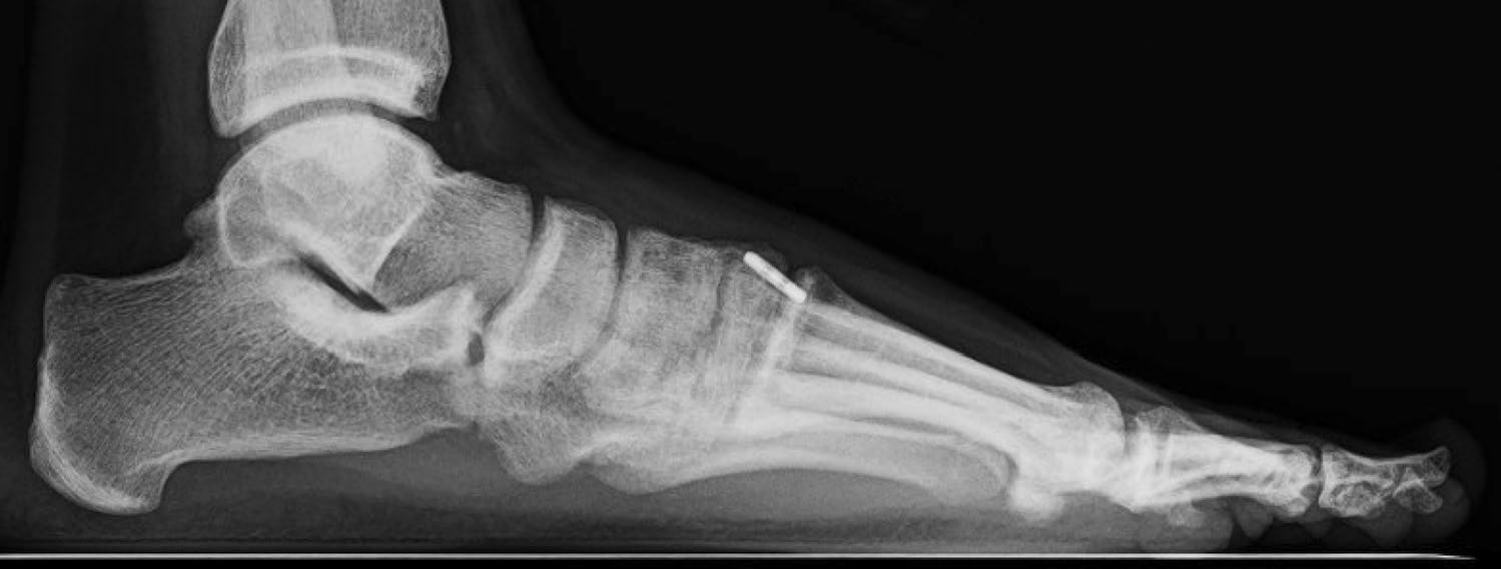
Postoperative lateral radiograph after Lisfranc IB application
